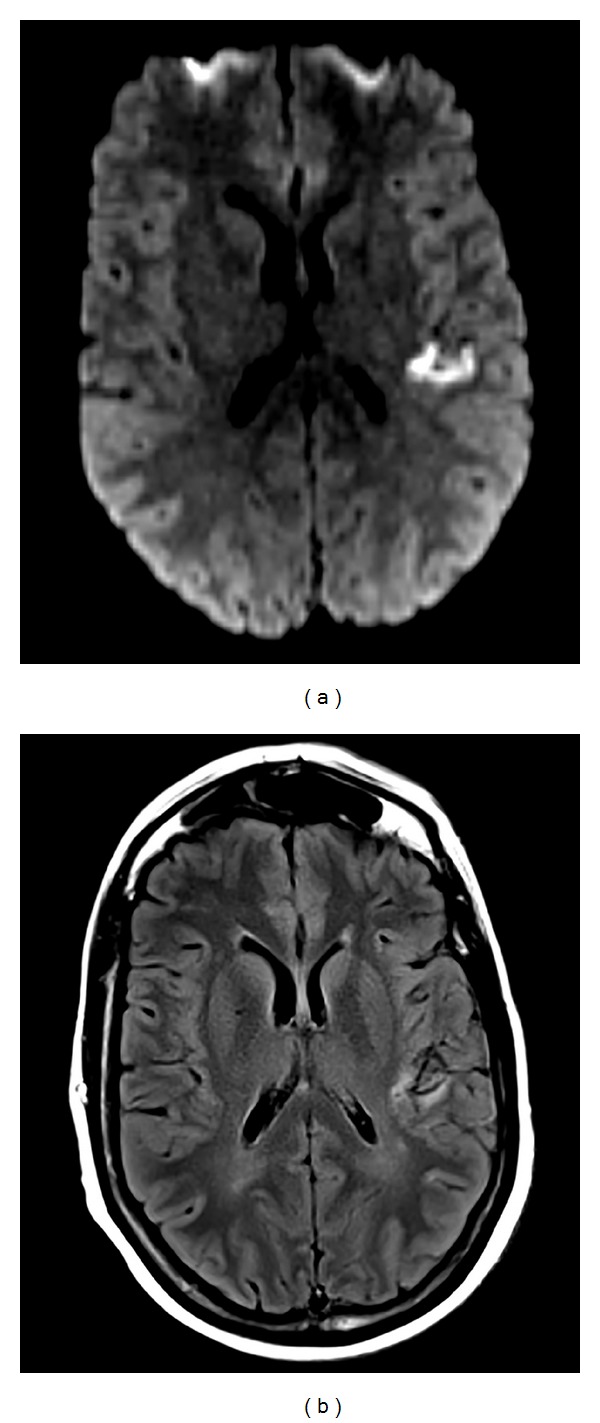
Magnetic resonance imaging (MRI) shows abnormal reduced diffusivity (a) and T2 hyperintensity (b) in the left posterior insula and temporoparietal operculum.
